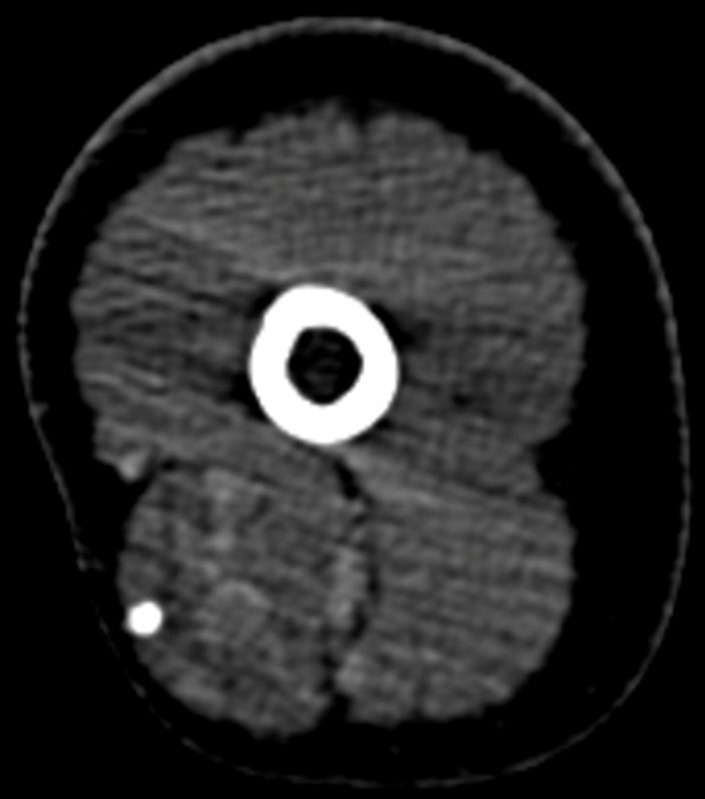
Post contrast CT 1 mm slice reconstruction in axial plane revealed the intramuscular tumors with calcification inside also with a lack of involvement of bone structures
